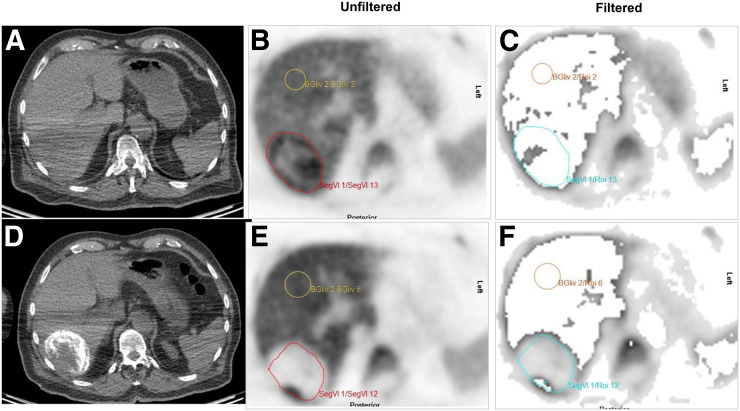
Axial CT, unfiltered ^18^F-FLT PET, and filtered ^18^F-FLT PET images before (A–C) and after (D–F) TACE show focal HCC lesion (red outline) with increased uptake at baseline and reduction after TACE. (B and E) In one of two baseline PET images before application of KSF (B), HCC is visible above background. (C) After application of KSF, tumor is mostly filtered out. (E) In post-TACE images, HCC is photopenic compared with surrounding liver before application of KSF. (F) After application, background liver activity is removed and HCC remains visible. BGliv = background liver; Seg = segment. Yellow outline indicates background liver, and blue outline indicates focal HCC lesion on following application of KSF.
